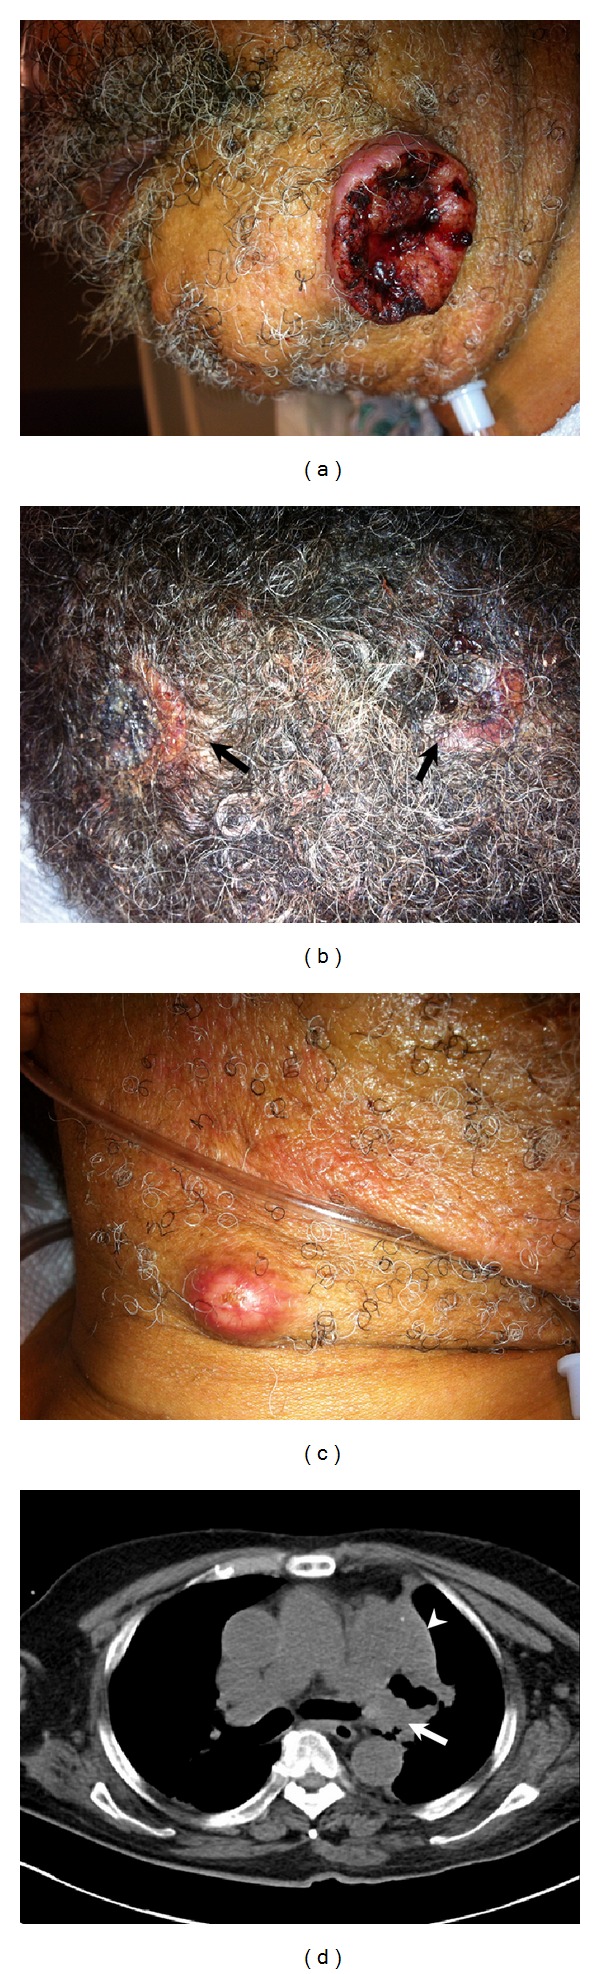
Skin finding revealed multiple large, firm, ulcerative skin lesions with hyperemic centers and elevated rolled edge over the left side of the chin (a) and the scalp (b). A nodular lesion also was seen on the right side of the neck (c). Computed tomography of the chest shows a 5 cm large centrally located left upper lobe mass (arrow) resulting in constrict of the left upper lobe bronchus and left upper lobe atelectasis (arrow head), associated with left hilar adenopathy.
